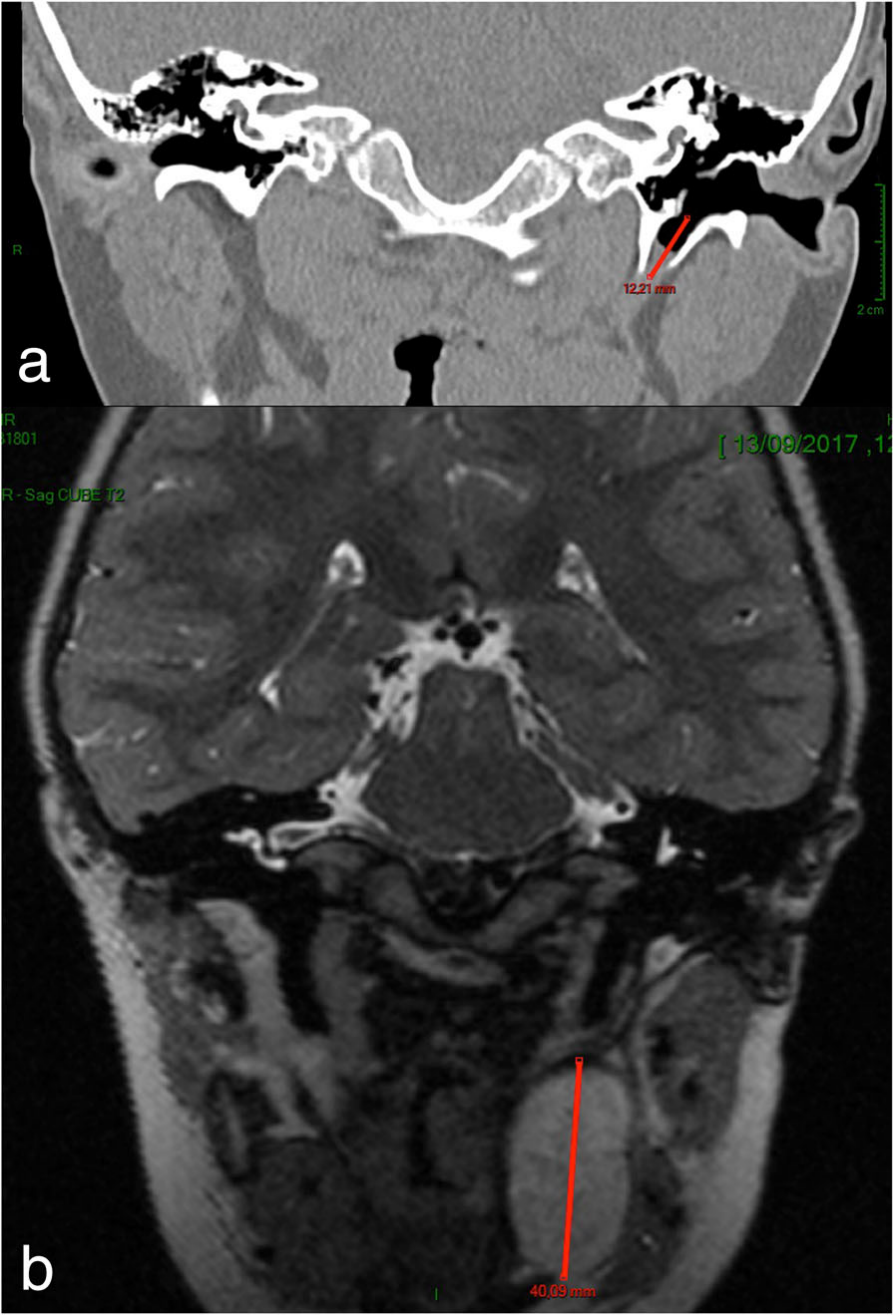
Imaging. a. Coronal CT scan and b. Coronal T2 weighted MRI key images demonstrating the bony canal lying medial to the facial nerve and a voluminous pharyngeal cyst
